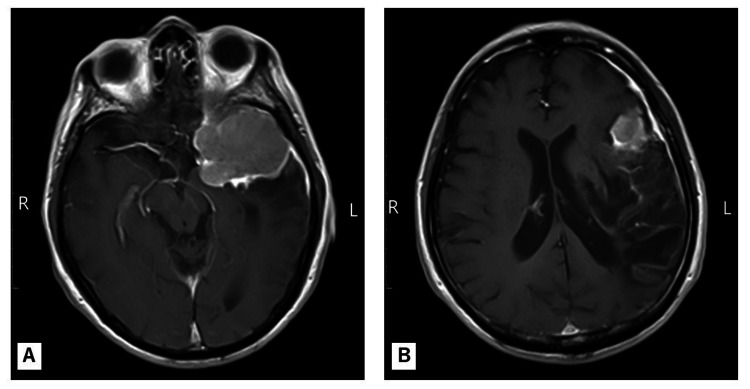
Gadolinium-enhanced axial T1-weighted brain MRI performed during the one-year follow-up (A) Homogeneous enhancement of a sphenoid ridge meningioma with a characteristic dural tail sign is observed. (B) The left frontal lobe is largely preserved, whereas the left parietal and temporal lobes show encephalomalacia and volume loss secondary to infarction.
